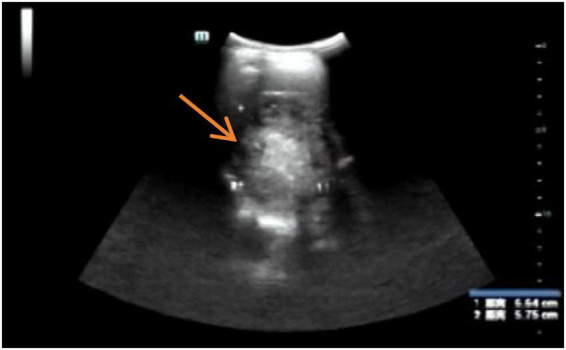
Five-month follow-up ultrasound. Ultrasonography obtained 5 months after embolization showed a residual mixed-echogenic lesion in hepatic segment VII without detectable internal Doppler flow, compatible with resolving post-hemorrhagic and post-embolization change. No sonographic evidence of recurrent hemorrhage or new perihepatic fluid collection was observed.
